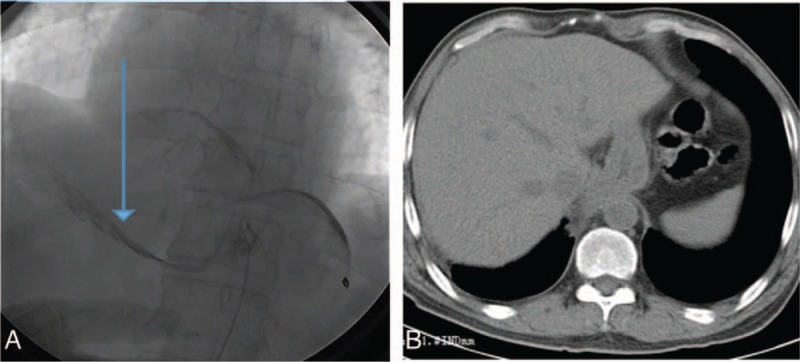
Emergency abdominal aortography (A) and CT (B) revealing remarkable extravasation of contrast medium that flowed out of the gastric tube. CT = computed tomography.
